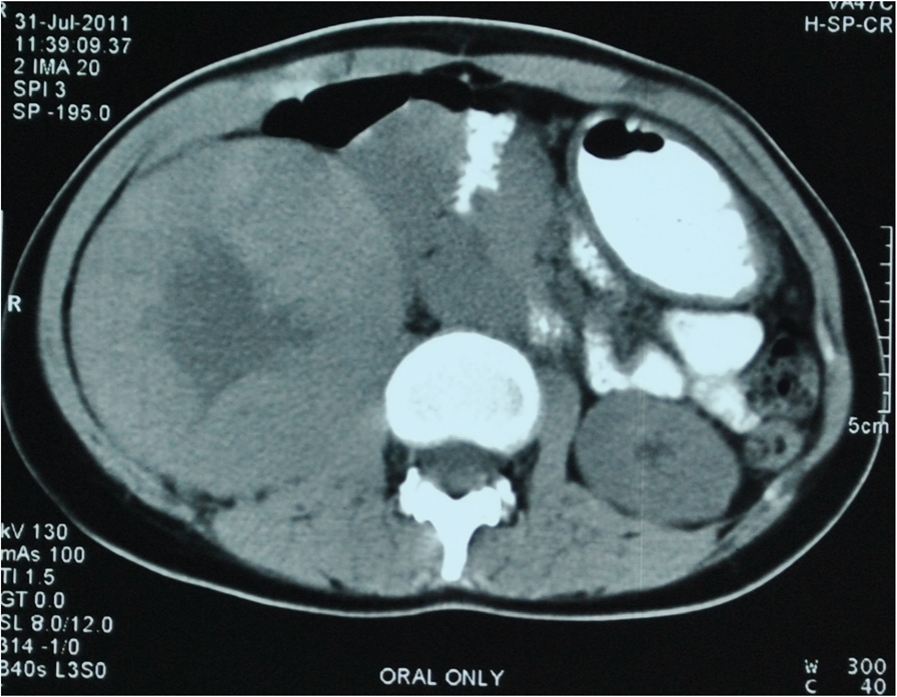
Computed tomogram showing large mass replacing almost whole of the right kidney with a functioning left kidney
